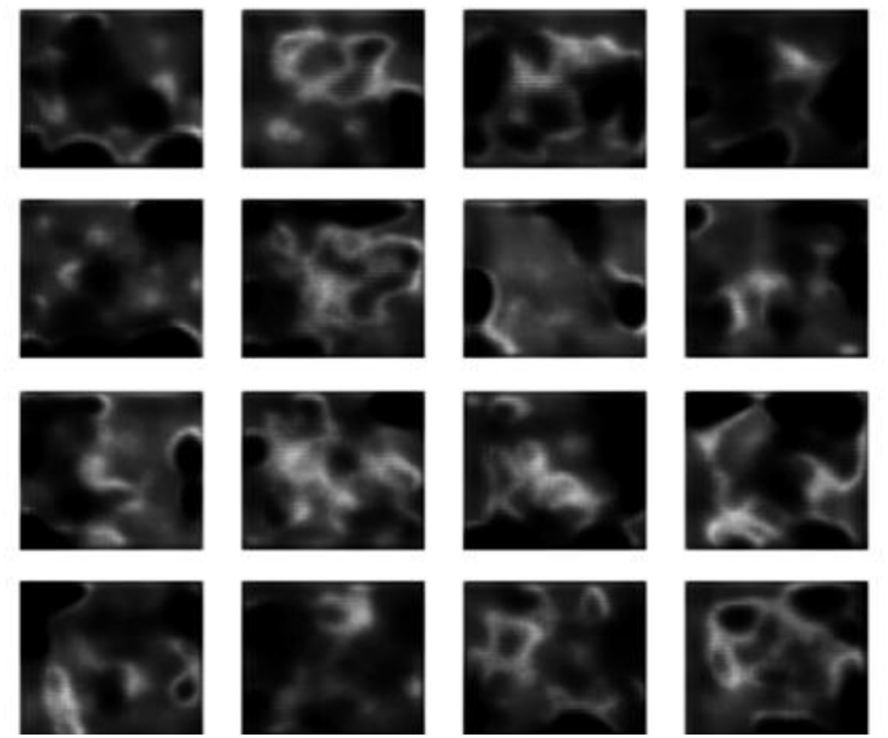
MRI generated by the baseline model.
